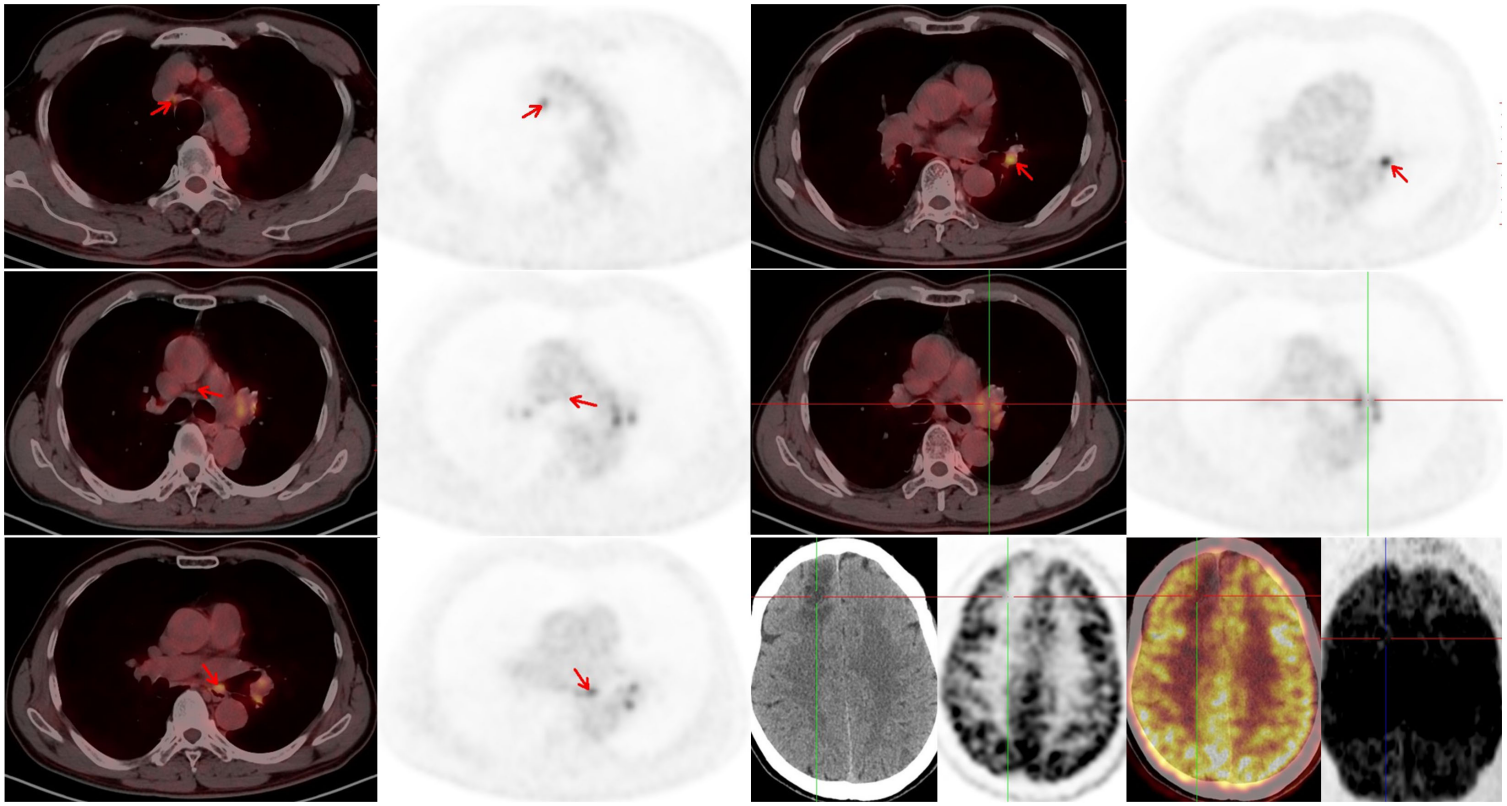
Positron emission tomography (PET)-computed tomography (CT) was performed on October 27, 2023. A residual tumor in the left lung hilar and mediastinal lymph nodes and new metastatic lesions in the brain can be observed.
